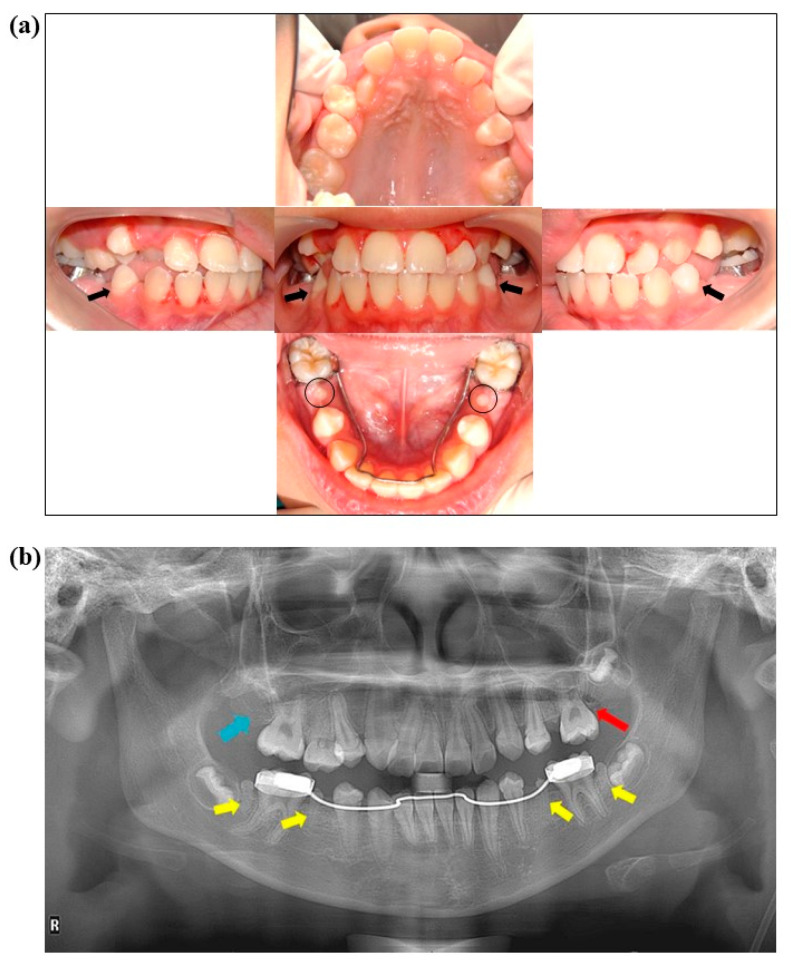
These images were taken of the patient at age 12, five years after completing dental treatment. (a) Intraoral pictures show microdontia on tooth numbers 35 and 45 (black circles). Black arrows show the cervical line on the enamel of tooth numbers 34 and 44 (chronological hypoplasia). A lingual holding arch was placed on the lower arch to prevent mesial shift of tooth numbers 36 and 46. (b) This panoramic radiograph was taken after the completion of dental treatment. It shows a DMFT of 0 on permanent teeth. The yellow arrows show microdontia on tooth numbers 35, 37, 45, and 47. The blue arrow shows where tooth number 17 is missing, and the red arrow shows a minor calcification mass on tooth number 27.
